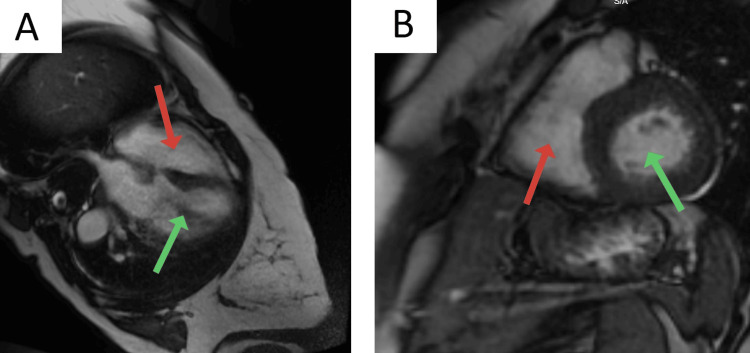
Cardiac Magnetic Resonance Imaging. A: Long axis. Red arrow: Right ventricle; Green arrow: Left ventricle B: Short axis. Red arrow: Right ventricle; Green arrow: Left ventricle
